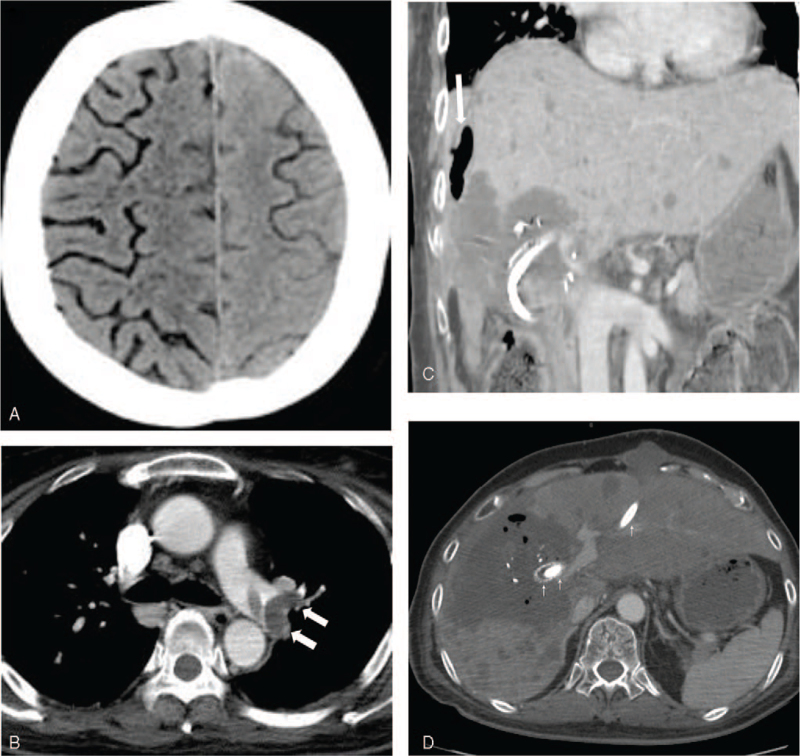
Massive cerebral air embolism after a series of PTBD tube placement in a 50-year-old woman with recurrent gallbladder cancer. Initial noncontrast brain CT shows extensive pneumocephali in the sulci of the right cerebral hemisphere with air densities in the superior frontal sulcus of the left frontal lobe. (B) Pulmonary embolism CT angiography reveals large filling defects in the left main and left lobar (upper and lower) pulmonary arteries arrows, consistent with pulmonary thromboembolism. (C) Coronal and (D) axial abdominopelvic CT shows a ruptured hepatic mass with free air and fluid in the perihepatic space (arrow) and air bubbles around the biliary stent (arrows) and PTBD tube (arrows).
